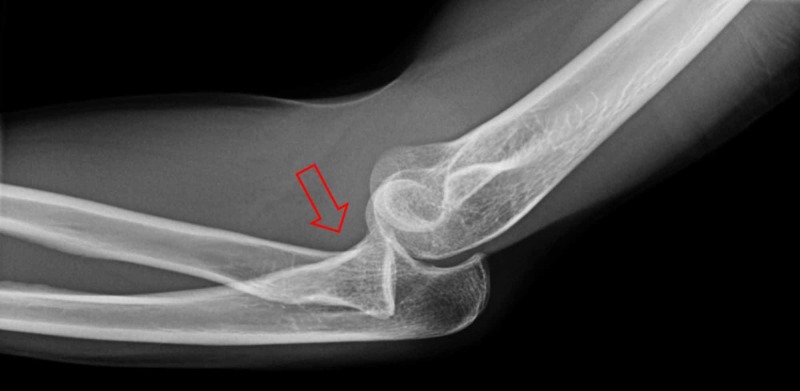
Pre-reduction lateral X-ray of the elbow showing a distal radial head dislocation with elbow subluxation
